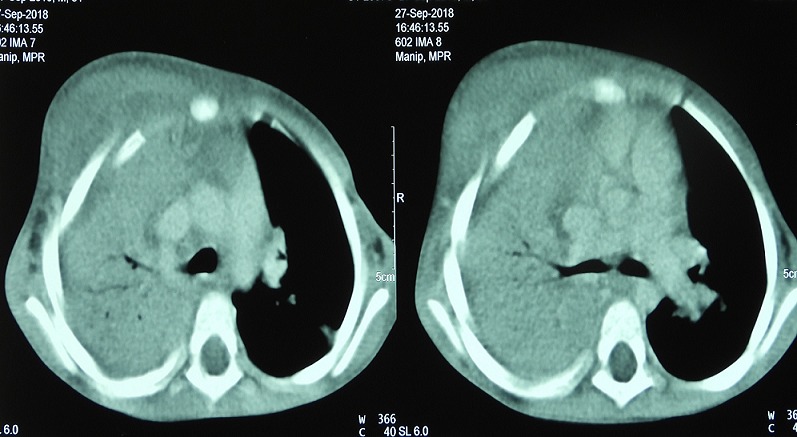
Contrast enhanced CT scans of the chest showing consolidative atelectasis of the right upper lobe with massive paratracheal adenopathy and chest wall mass. Enlarged axillary lymph nodes and erosion of the ribs are also noted
